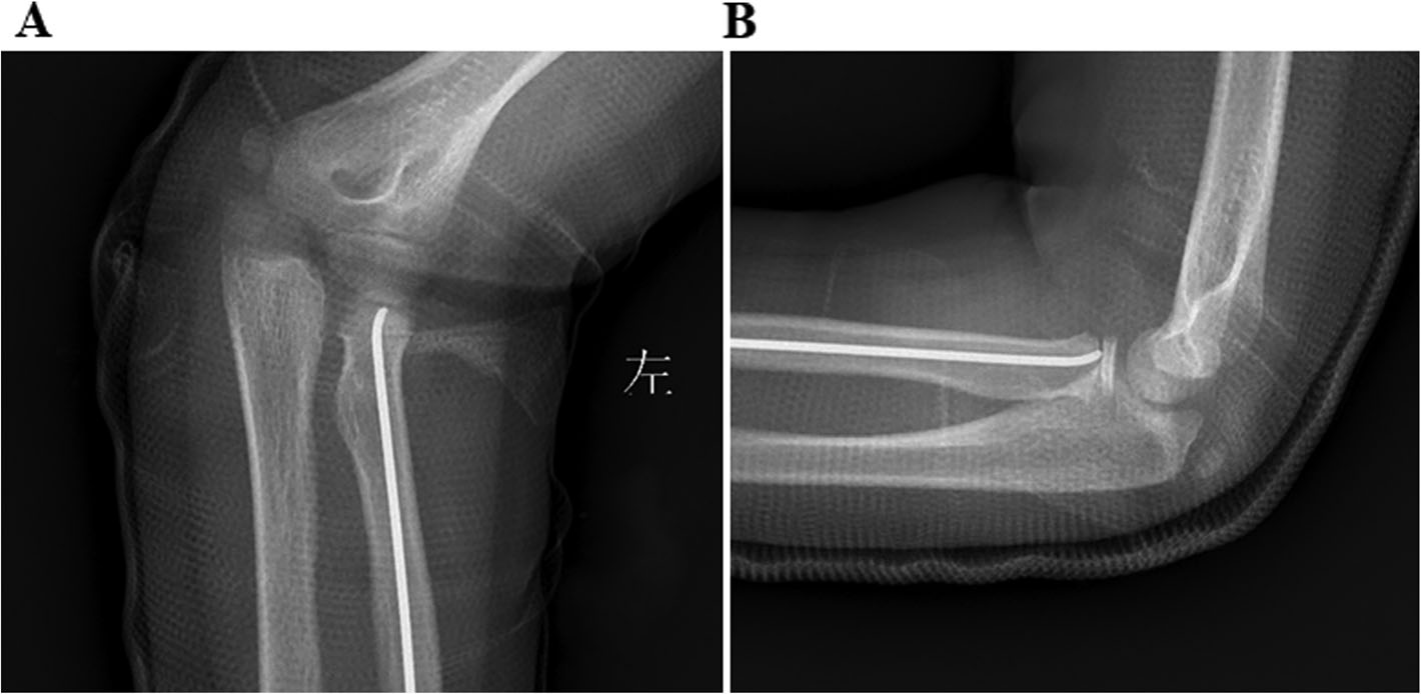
Postoperative AP and lateral X-ray of patient No. 20
